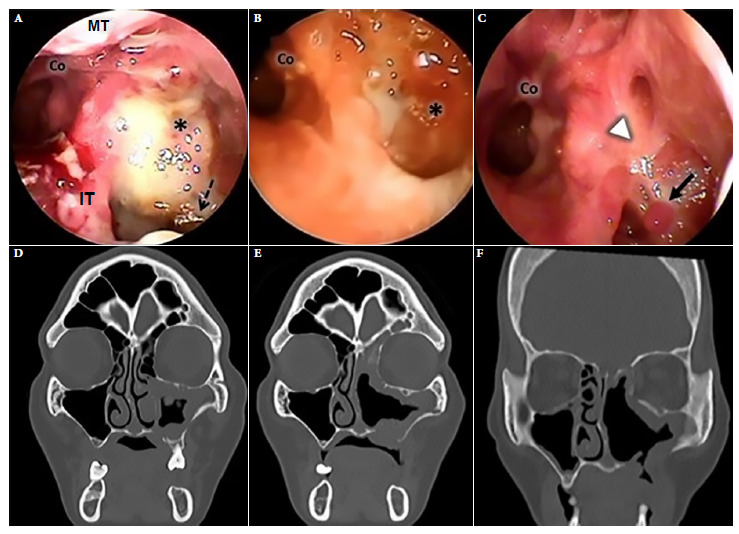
Case 1. Left nasal endoscopy. (A) Admission endoscopy (B) Endoscopy during standard treatment. (C) Endoscopy after 30 sessions of treatment with adjuvant hyperbaric oxygen. Facial CT scan. (D) On admission, hypertrophy of the mucosa of the left maxillary sinus, bone lysis of its medial and inferior wall and palatine bone. Obstruction of the left ostiomeatal complex. (E) CT scan during standard treatment. (F) Tomographic control after 30 sessions of treatment with adjuvant hyperbaric oxygen, shows improvement of the nasal mucosa and oroantral fistula. Asterisk: posterior wall of maxillary sinus, Co: choana, S: septum, IT: inferior turbinate, MT: middle turbinate; dashed black arrow: devitalized bone tissue, white arrowhead: scar mucosa, continuous black arrow: granulation tissue.
